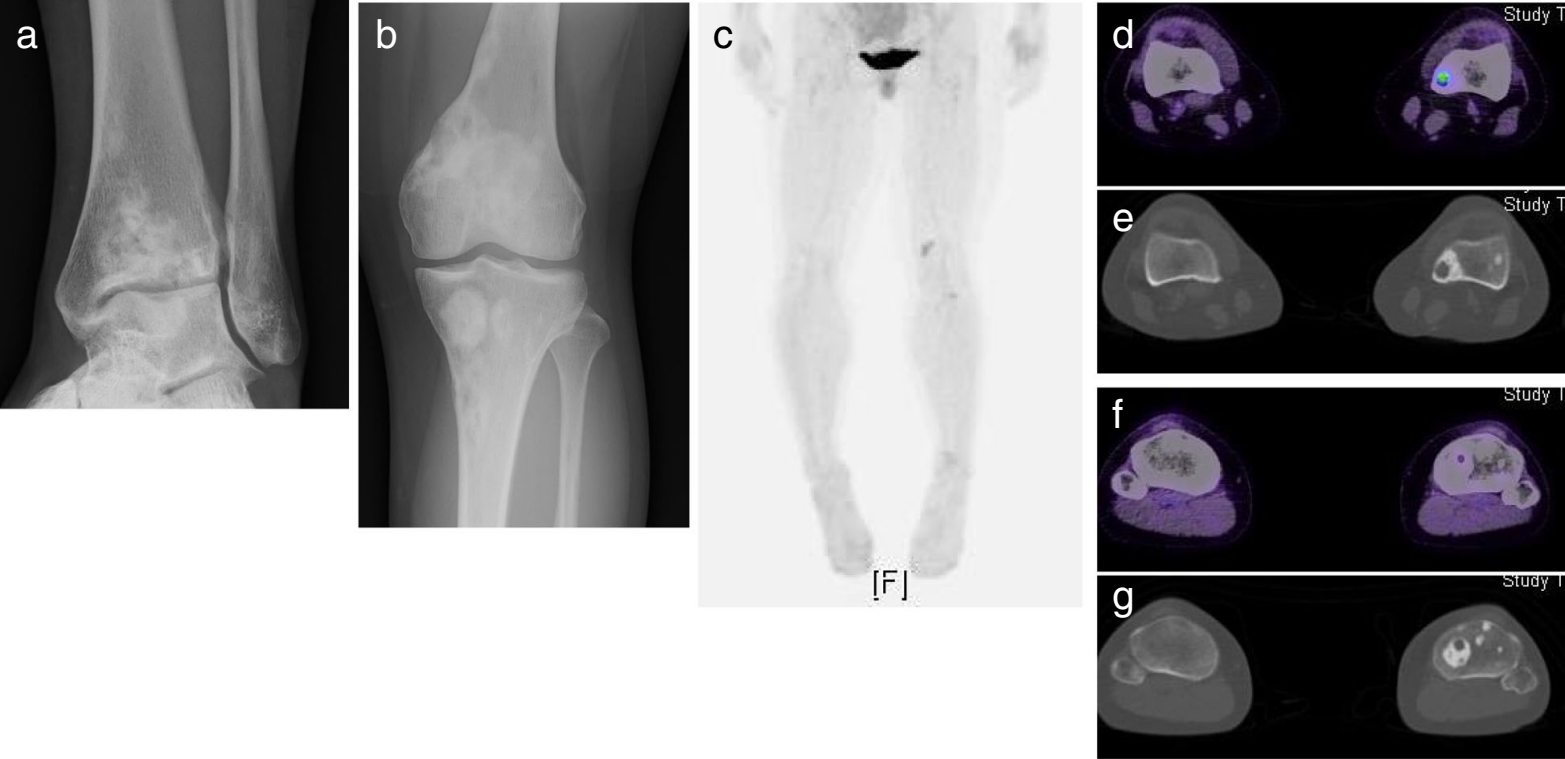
Imaging findings 4 years following denosumab treatment. a and b Plain radiographs showed shrinkage of the lesions with striking marginal sclerosis. c-g Fluorodeoxyglucose (FDG)-positron emission tomography/computed tomography showed that each lesion had become less FDG-avid with a maximum standardized uptake value of 2.6 and was accompanied by remarkable sclerotic bone
